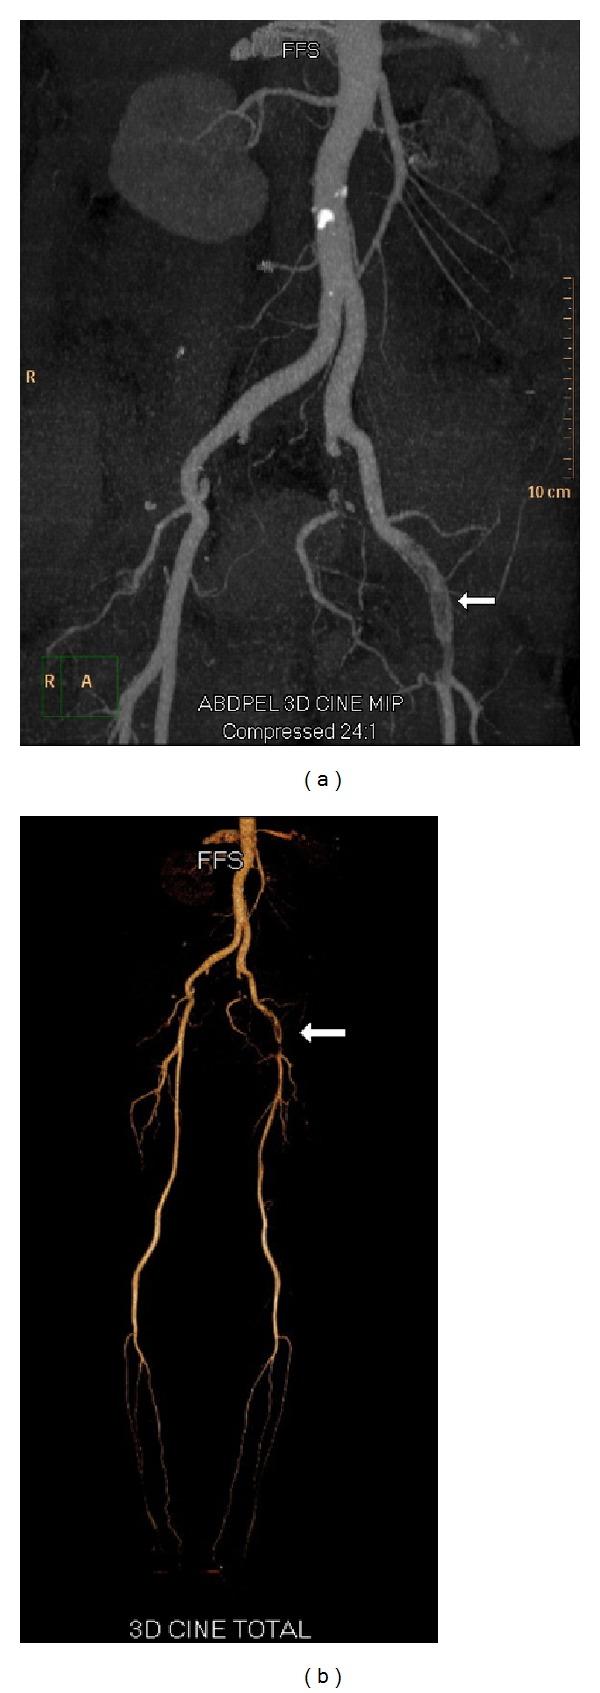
CTA of bilateral lower extremities. White arrow points to filling defect of left external iliac artery.
